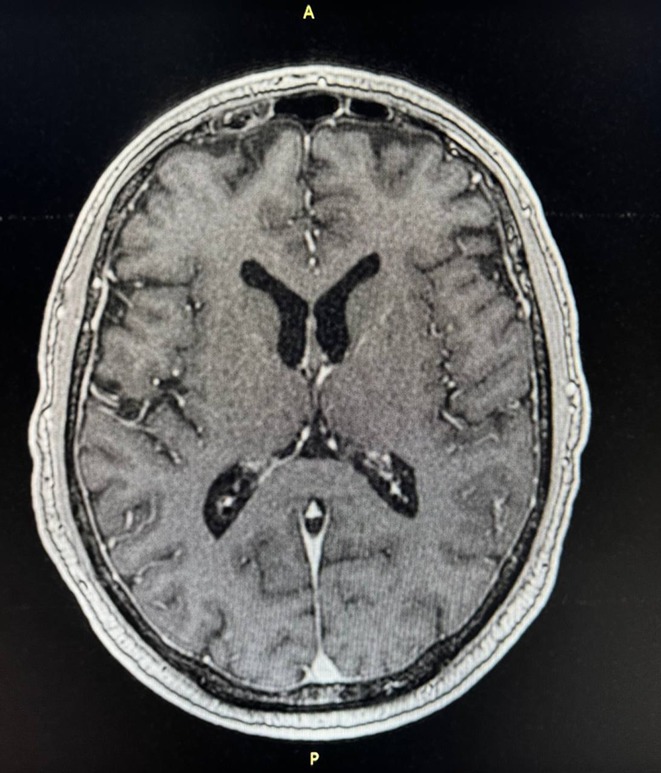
FIGURE 1 Patient's brain MRI T1 post contract sequence, axial projection ‐leptomeningeal post‐contrast enhancement.
